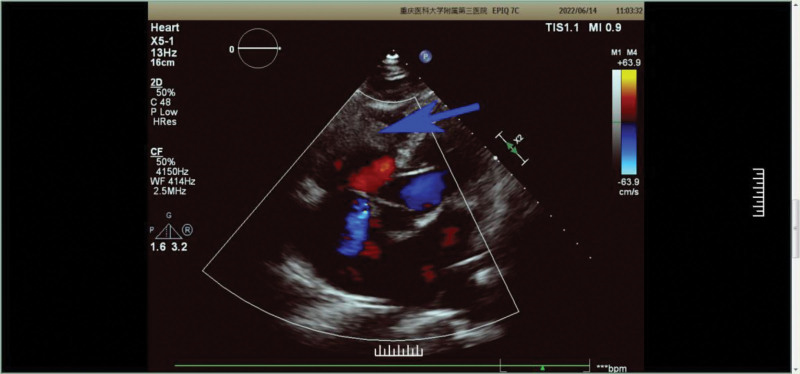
Echocardiography on June 14th: the location indicated by the blue arrow indicates the possibility of a tumor occupying lesion.
